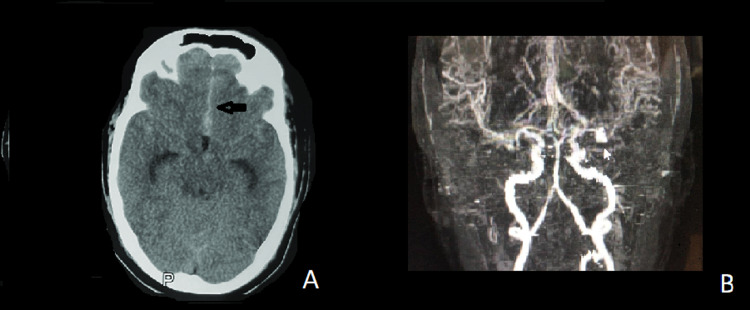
(A) Axial slide of plain CT brain showing nonaneurysmal SAH into the anterior interhemispheric fissure and dilated both temporal horns of lateral ventricles. (B) CT angiography showing no vascular abnormality in the anterior circulation.
